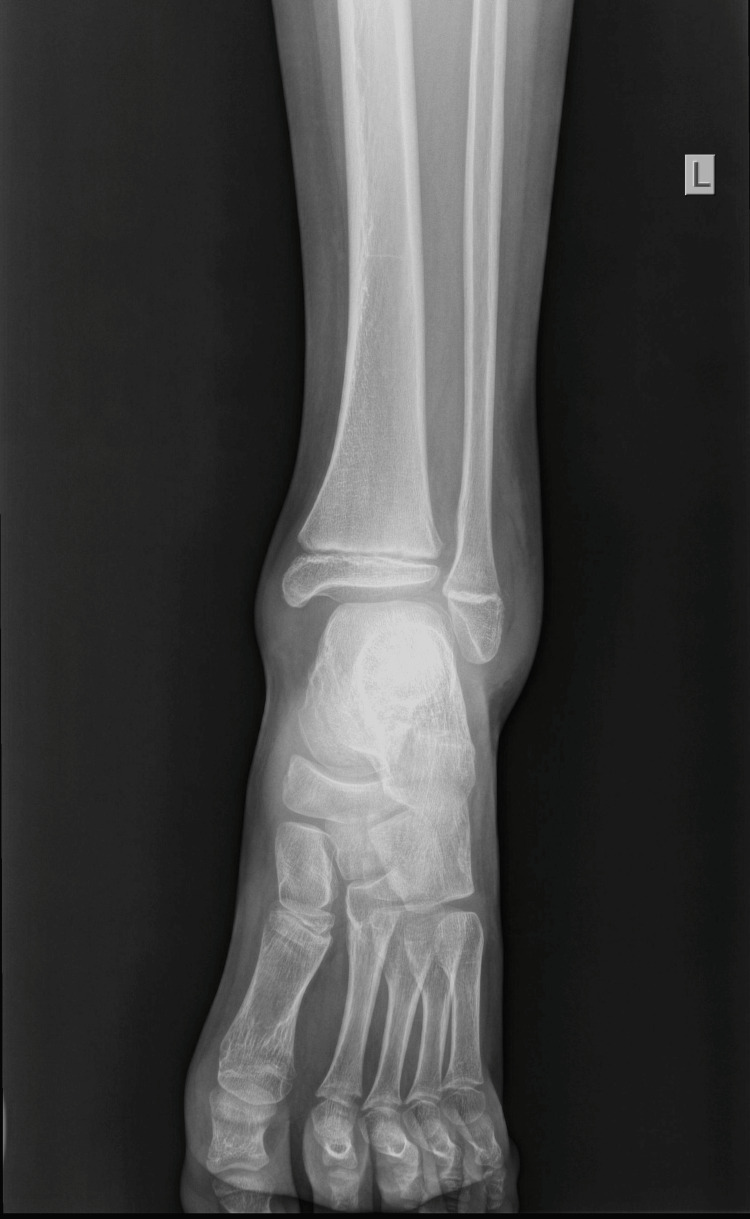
Initial X-ray AP diagnosed as an ankle sprain, with soft tissue oedema of the lateral malleolus.
